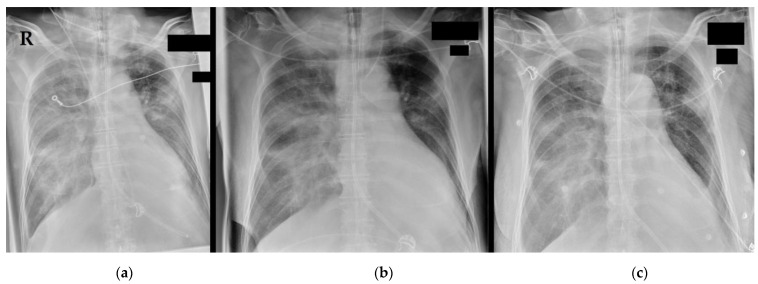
Chest X-ray at admission to the emergency department: Chest X-rays at (a) admission, (b) +14 h, and (c) +34 h. The endotracheal tube was detectable cranial of the carina, a gastric tube was placed, and from (b), a central venous catheter was inserted into the left internal jugular vein. Bilateral consolidations are compatible with pneumonic infiltrations and congestion. Bilateral pleural effusions can unfortunately not be optimally described due to the condition of the shot. The left upper lateral lobe shows the known bronchial tumour. R = right patient side.
